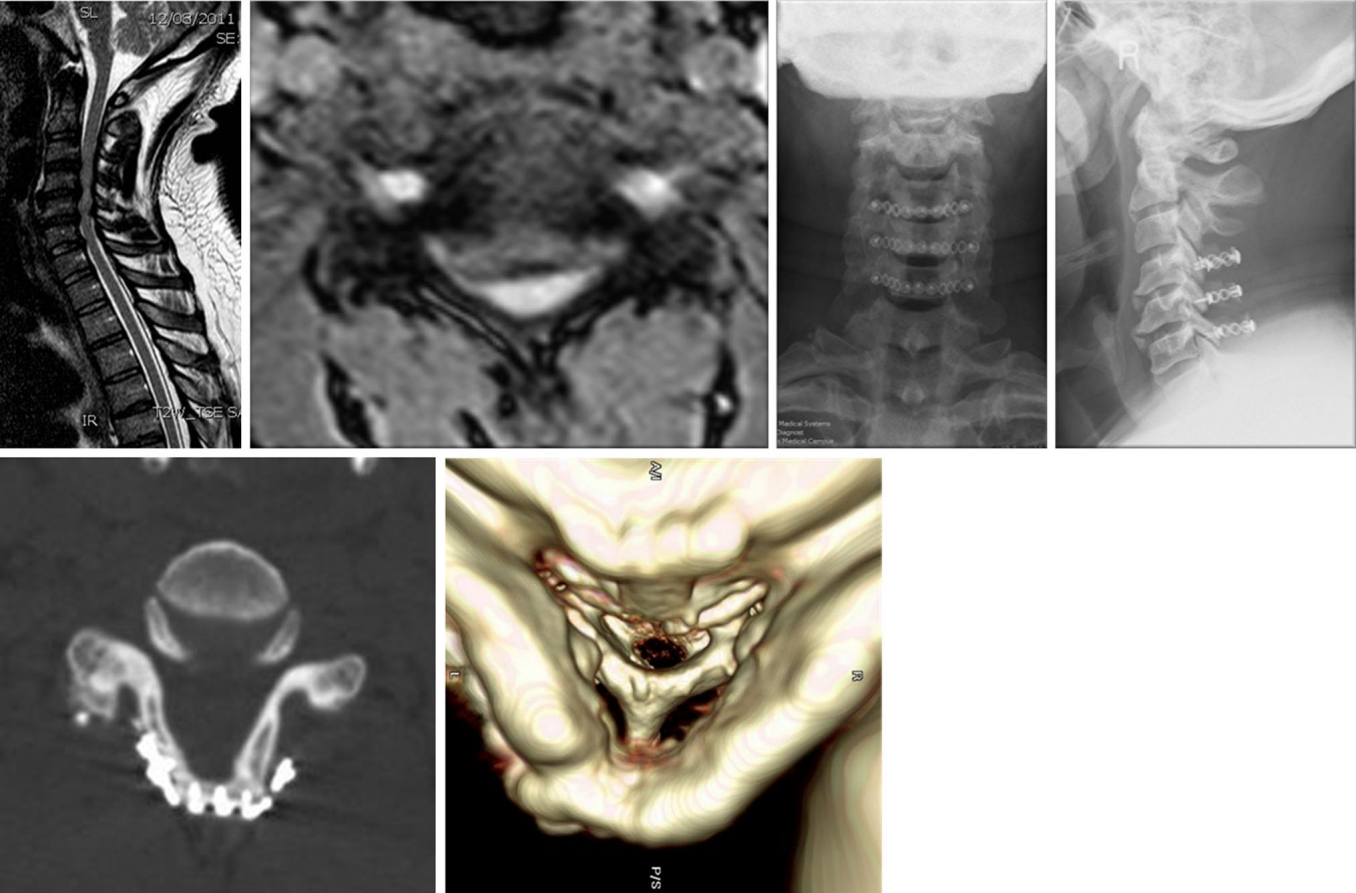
A case example of our modified hardware-augmented French-door laminoplasty technique. Sagittal and coronal views of preoperative MRI are shown with postoperative X-ray of the cervical spine. CT scan analysis at 1 year after the operation shows good decompression of the spinal canal and graft fusion
